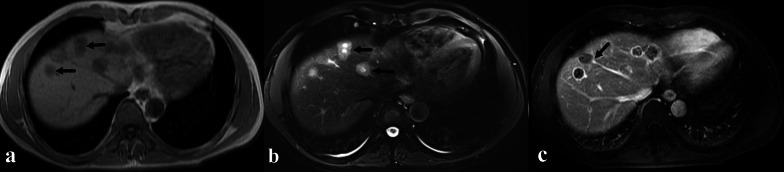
A 42-year-old male HEH patient. a Coalescent lesion with target sign on T1-weighted 3D GRE image, consisted of concentric layers including markedly hypointense in central tumor regions and peripheral zones which were moderately hypointense relative to liver parenchyma (arrows). b Coalescent lesion with target sign on fat-saturated T2-weighted TSE image, consisted of concentric layers including markedly hyperintense in central tumor regions and moderately hyperintense peripheral zone (arrows). c Lollipop sign on portal phase image, consisted of a well-defined tumor (as the “candy”) and an obstructed hepatic vein (as the “stick”) (arrow)
